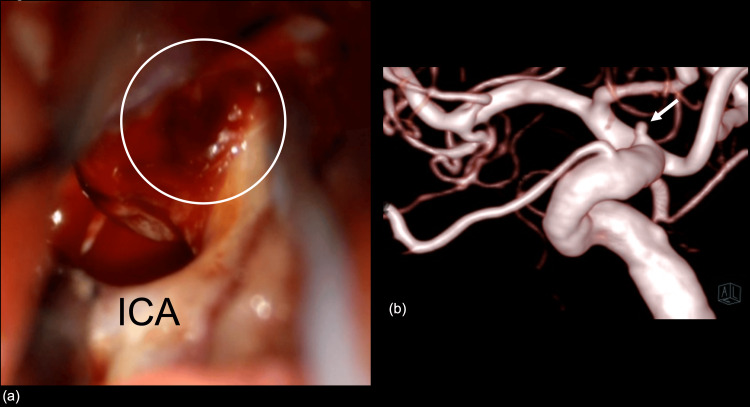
Intraoperative photograph and follow-up computed tomography angiography An intraoperative photograph shows the blood blister-like aneurysm on the right internal carotid artery (Figure 4a). The enlarged aneurysm was identified on the follow-up computed tomography angiography (Figure 4b), and the patient underwent endovascular treatment with stent-assisted coil embolization. ICA: internal carotid artery
